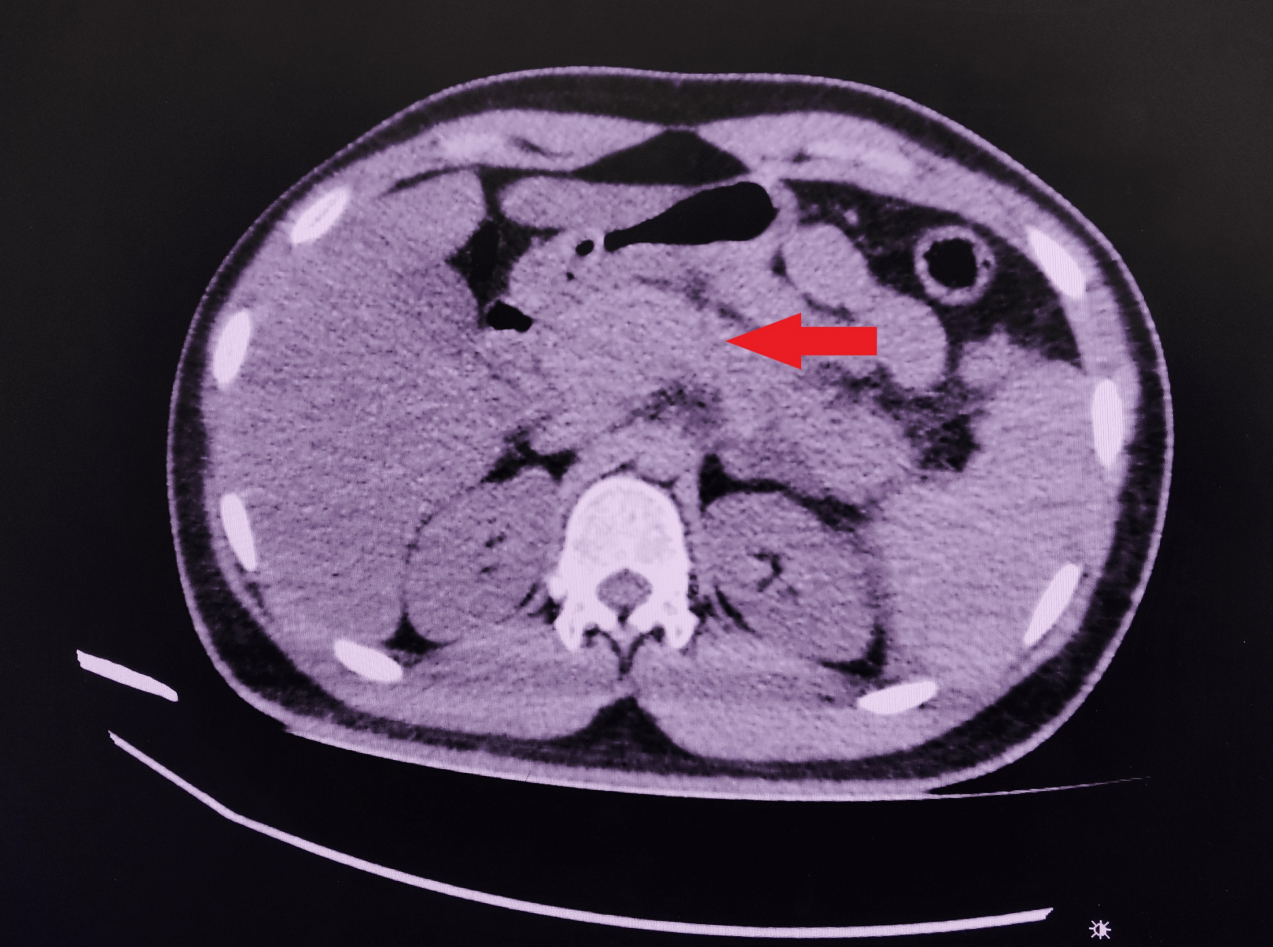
CT scan axial cut showing bulky pancreas with peripancreatic fat stranding (red arrow) CT: computed tomography
